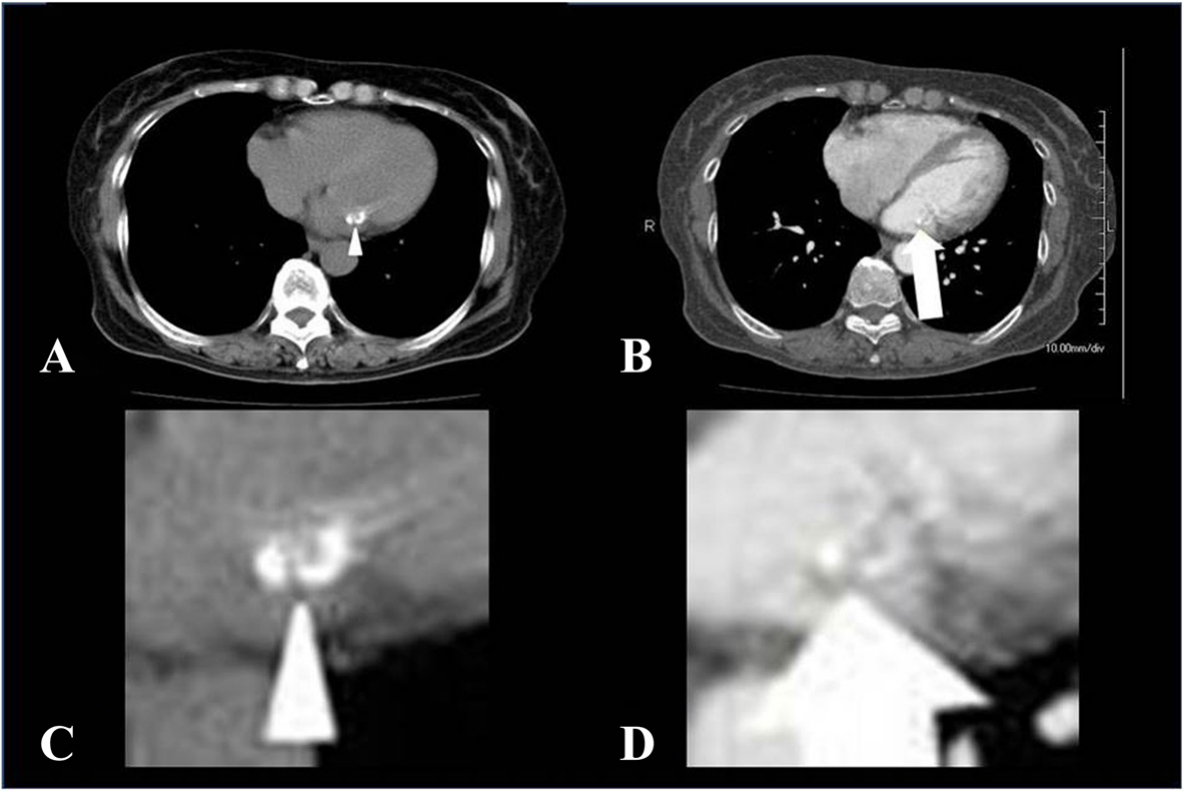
Computed tomograms (CT) of the heart. CT of the heart without contrast medium revealed a calcified mass with a mixture of high and low density area along the posterior mitral leaflet (arrow head) (A), and another computed CT with contrast medium demonstrated a hypodensity central part of the mass (arrow) (B). The zoomed CT of the heart without contrast medium revealed a calcified mass with a mixture of high- and low-density area along the posterior mitral leaflet (arrow head) (C), and another zoomed CT with contrast medium demonstrated a hypodensity central part of the mass (arrow) (D).
